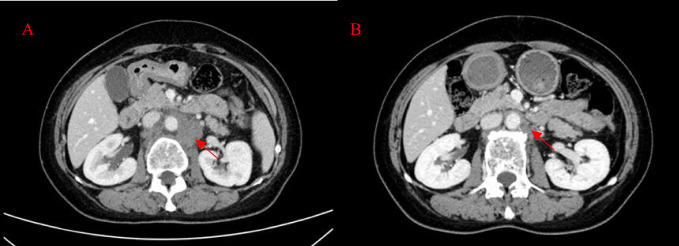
Patient’s CT imaging before and during T-DXd treatment. (A) The para-aortic lymph nodes were enlarged (2022-5-13); (B) The para-aortic lymph nodes decreased after T-DXd treatment (2022-6-29).
